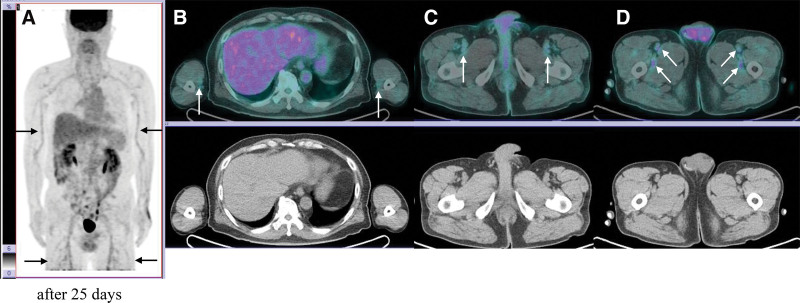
The second F-18 FDG PET/CT after prednisolone treatment. Follow-up F-18 FDG PET/CT performed 25 d after the initial scan showed significantly decreased metabolic activity in the previously affected arteries (A–D), indicating a favorable treatment response. Arrows indicate the involved arteries. CT = computed tomography, FDG = fluorodeoxyglucose, PET = positron emission tomography.
